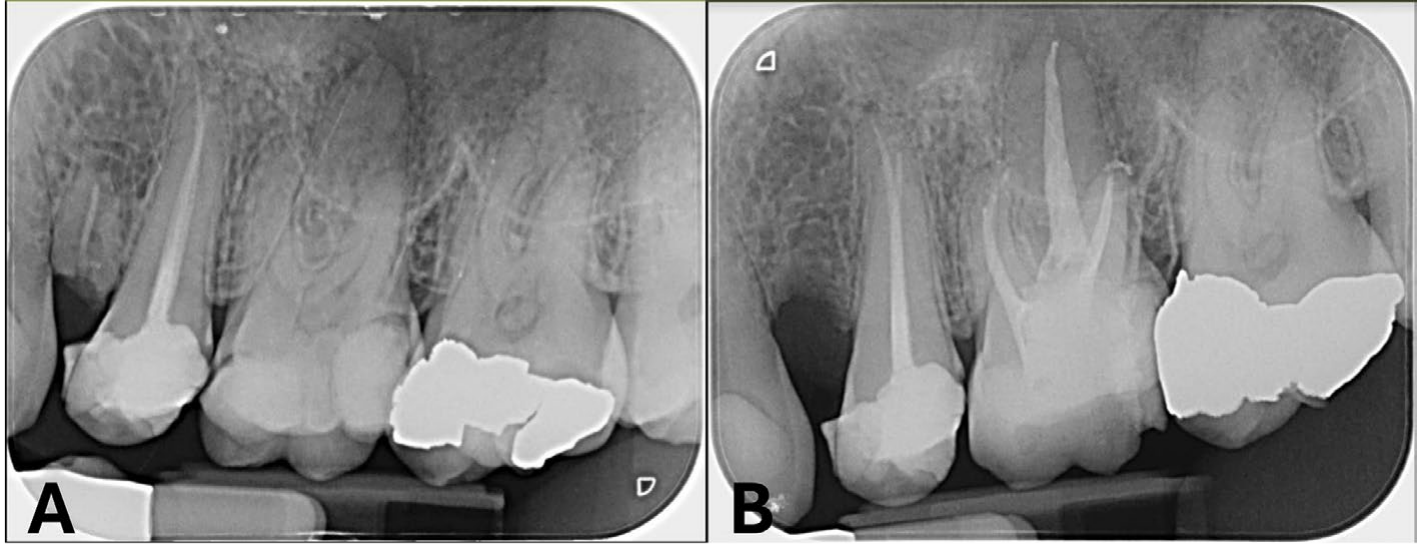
a) Preoperative periapical radiograph of left maxillary first molar with symptomatic apical periodontitis. b) Postoperative radiograph showing root canal treatment achieving technical operative factors determined by Ng *et al.* 2008 which significantly improve the outcome of primary root canal treatment. Case displayed was carried out by Shruti Panchani (undergraduate student at Birmingham School of Dentistry)
